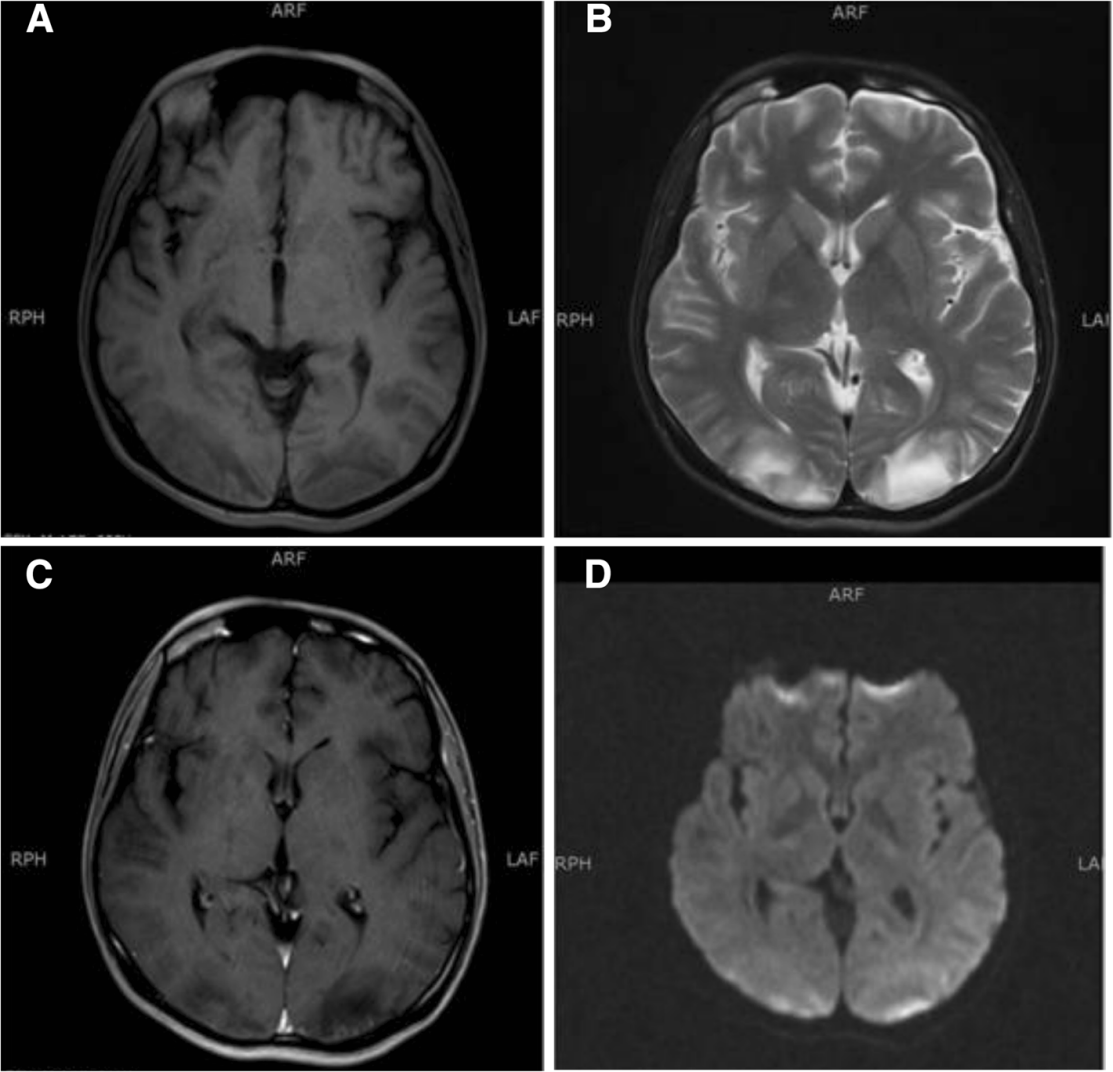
Posterior reversible encephalopathy syndrome after cisplatin treatment. Bilateral and symmetrical abnormal intensity areas are identified in the occipital lobes involving the cortical and subcortical locations. These are hypointense on T1-weighted images (A) and hyperintense on T2-weighted images (B), showing minimal patchy post-contrast enhancement (C) with no diffusion restriction (D).
